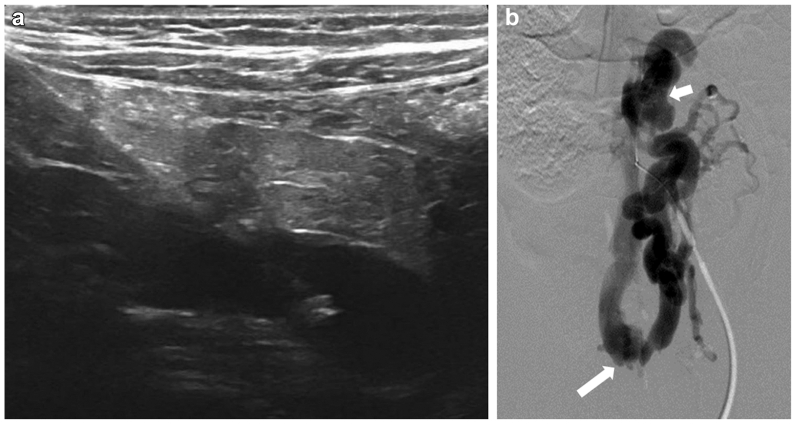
Patient 2 had a history of portosystemic shunt requiring portal pressure measurement before surgical ligation. (A) Ultrasound image depicting the needle placed via the abdominal wall into dilated inferior mesenteric vein. (B) Portomesenteric venography showing dilated inferior mesenteric vein (*short arrow*) with retrograde flow to the right internal iliac vein (*long arrow*).
